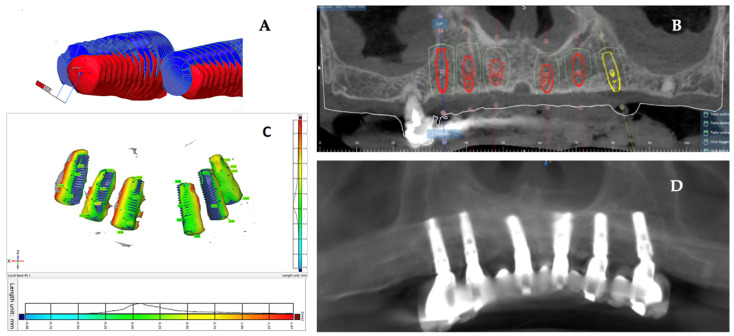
Data analysis: (A,C) superimposition of implant planning and postoperative CBCT images. It is possible to graphically see the discrepancy between the inserted and planned implants; (B,D) preoperative CBCT images with virtually inserted implants used for fabrication of surgical template; image of the postintervention control CBCT.
